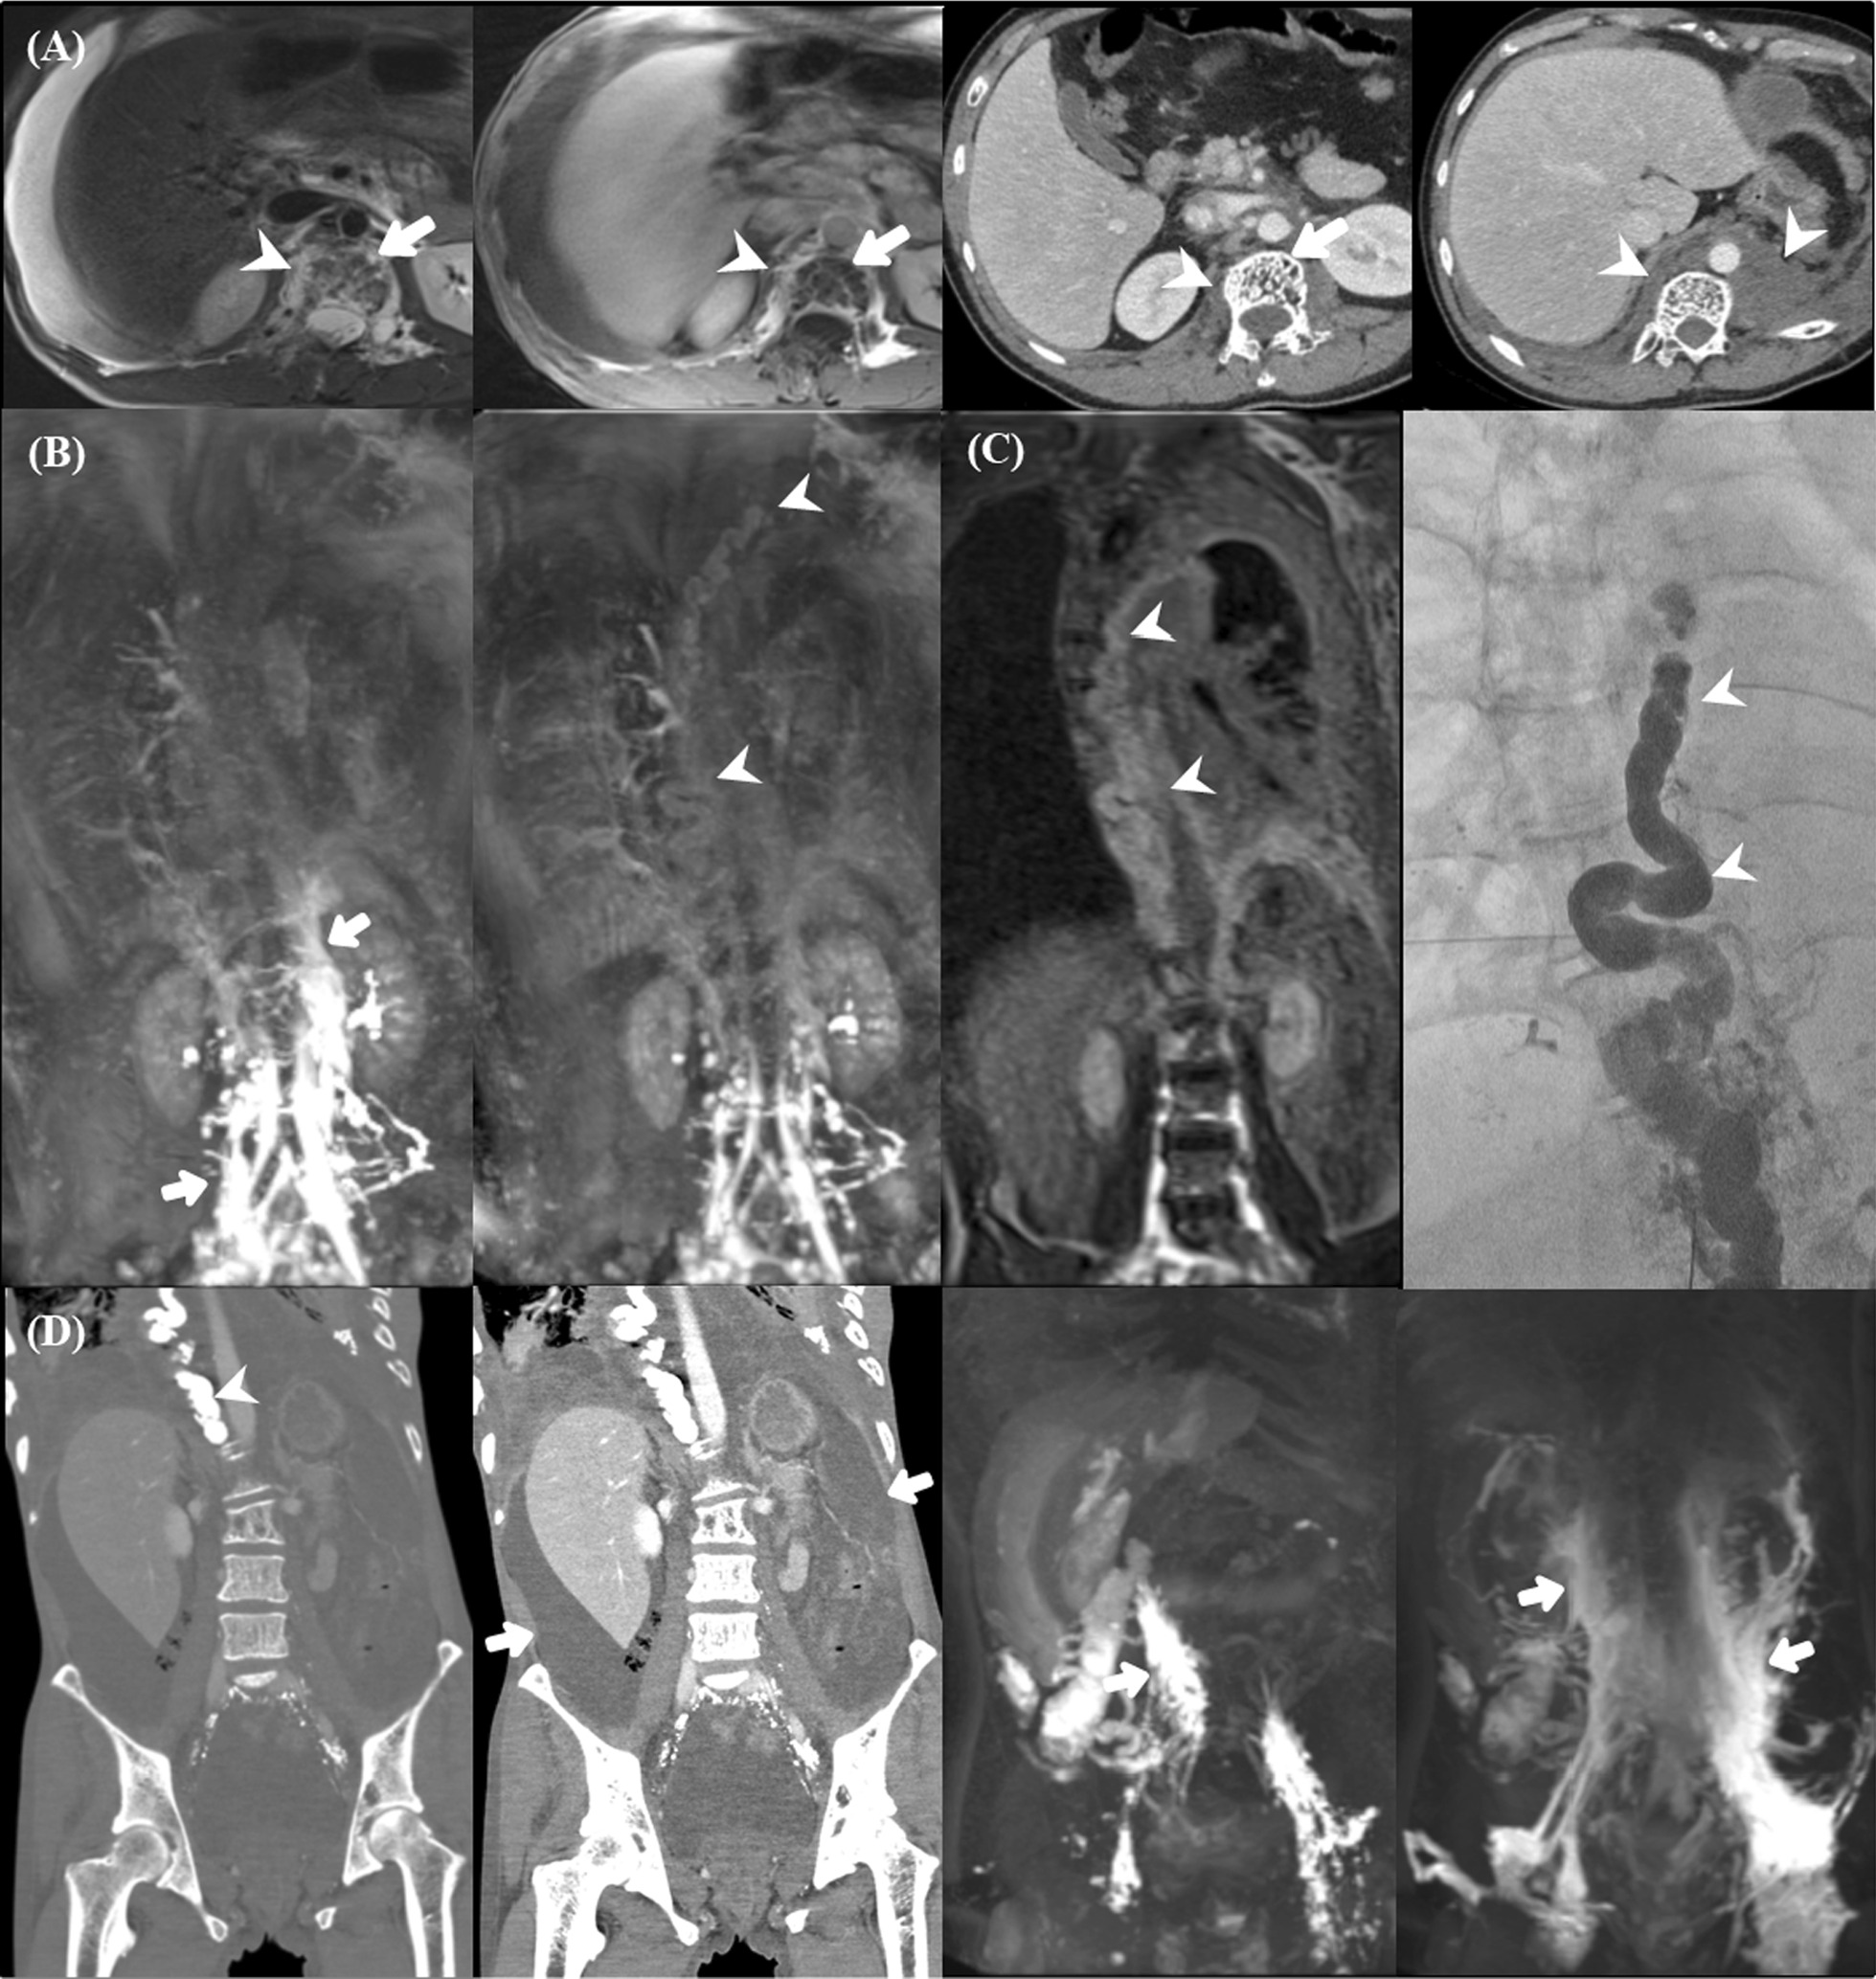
A 31-year-old male with Gorham–Stout disease. A 31-year-old man diagnosed with Gorham‒Stout disease presented with dyspnea related to massive chylous pleural effusion on the right side. Dynamic contrast-enhanced magnetic lymphangiography (DCMRL) consisted of axial T2-weighted image (T2WI), axial/coronal contrast-enhanced T1-weighted image (T1WI), and maximum intensity projection (MIP) reconstruction images. A T2WI (first column) and contrast-enhanced T1WI (second column) reveal multiple bundles of hypertrophied lymphatic vessels with a dark signal in the thickened right posterior parietal pleura and contrasting bright signals (arrowheads), and there is a contrast-enhancing vertebral lesion (arrows). Contrast CT (third and fourth columns) showed osteolytic changes (arrow) and retroperitoneal soft-tissue lesions (arrowheads). B MIP images (first and second columns) showing extensive collateral lymphatic vascular channels in the retroperitoneal space (arrows) and a faint giant thoracic duct (arrowheads). C A faintly filled, large abnormal thoracic duct is visible on the coronal T1WI before MIP reconstruction (first column, arrowheads). The giant thoracic duct is confirmed using digital subtraction angiography (second column, arrowheads). D After thoracic duct embolization (first column, arrowheads), the right pleural effusion was controlled, but uncontrolled ascites developed (second column, arrows), and the patient returned to the hospital one year later. When DCMRL was performed again, lymphatic flow (third and fourth columns, arrows) in the abdominal cavity was enriched, which was thought to have occurred because of the blocked outlet
